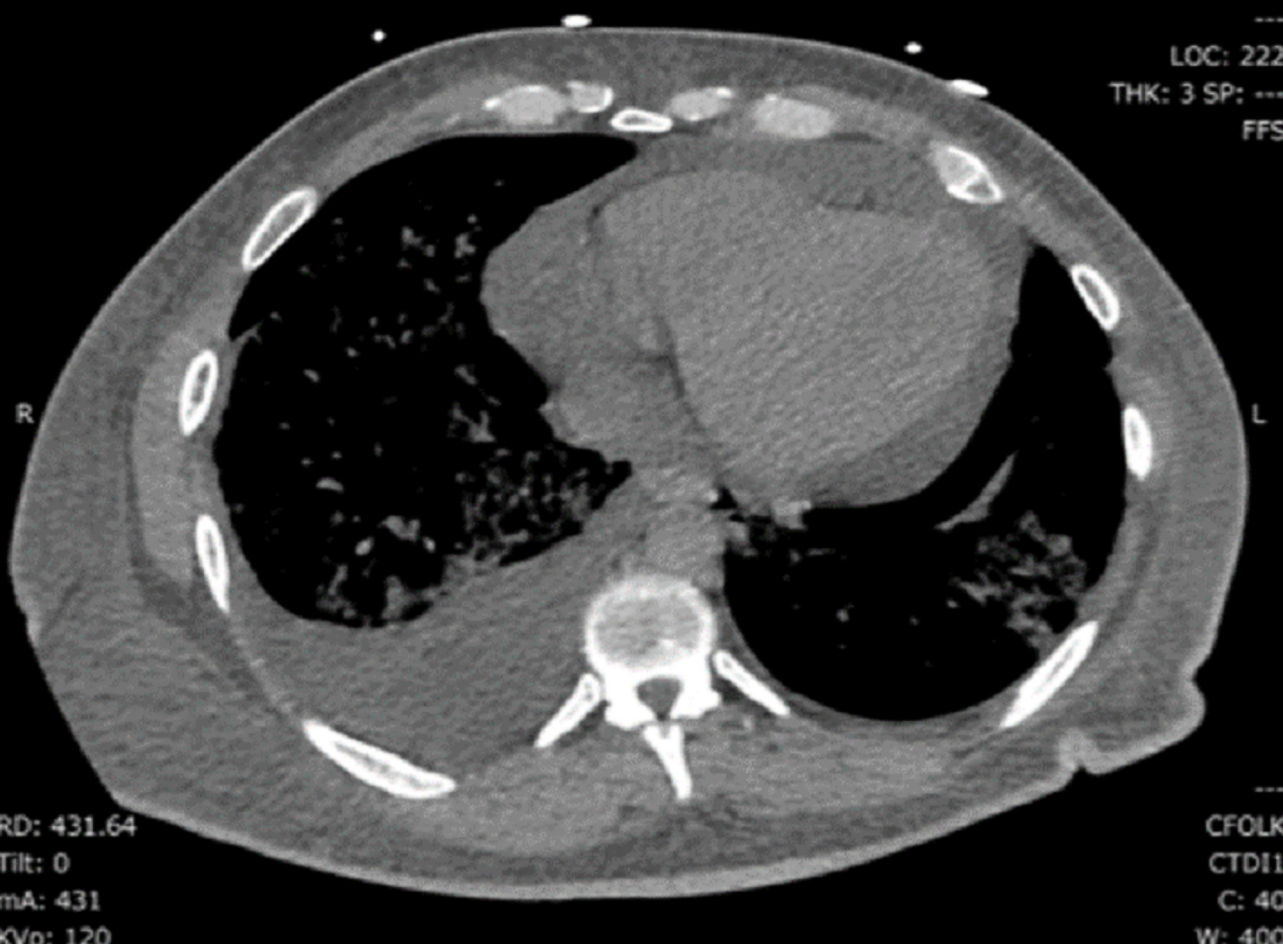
Computed tomography of the chest. Mediastinal window showing pericardial and pleural effusion (Day 3)
